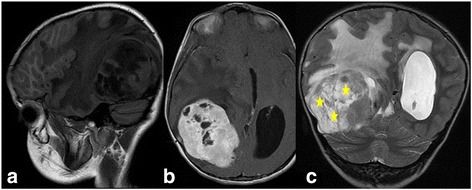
Cranial magnetic resonance imaging revealed a right, occipito-temporal, voluminous, well-demarcated mass, hypointense heterogenous on T1-weighted images (a) with a strong contrast enhancement (b) and a characteristic multicystic bubbly appearance on T2-weighted images (c). There was a peritumoral edema and a monoventricular left hydrocephaly. The stars indicate the tumor
